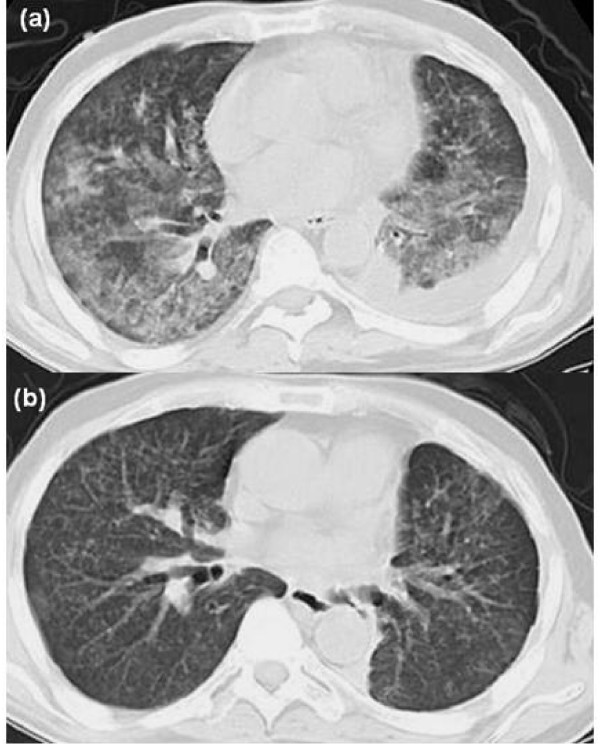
Radiological evaluation of gefitinib-related interstitial lung disease on CT scans. (a) before treatment, (b) after treatment.
